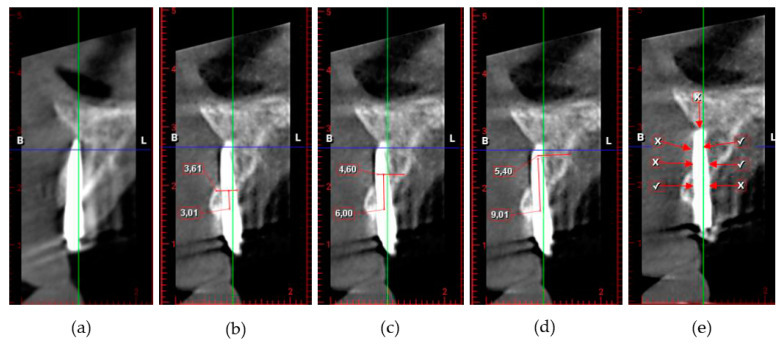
(a) CBCT cross sectional view of MDI placed in location 23; (b) measurement of bone thickness at 3 mm; (c) measurement of bone thickness at 6 mm; (d) measurement of bone thickness at 9 mm; (e) perforations marked with (X) and bone-to-implant contact (BIC) buccal/palatal marked with (V).
